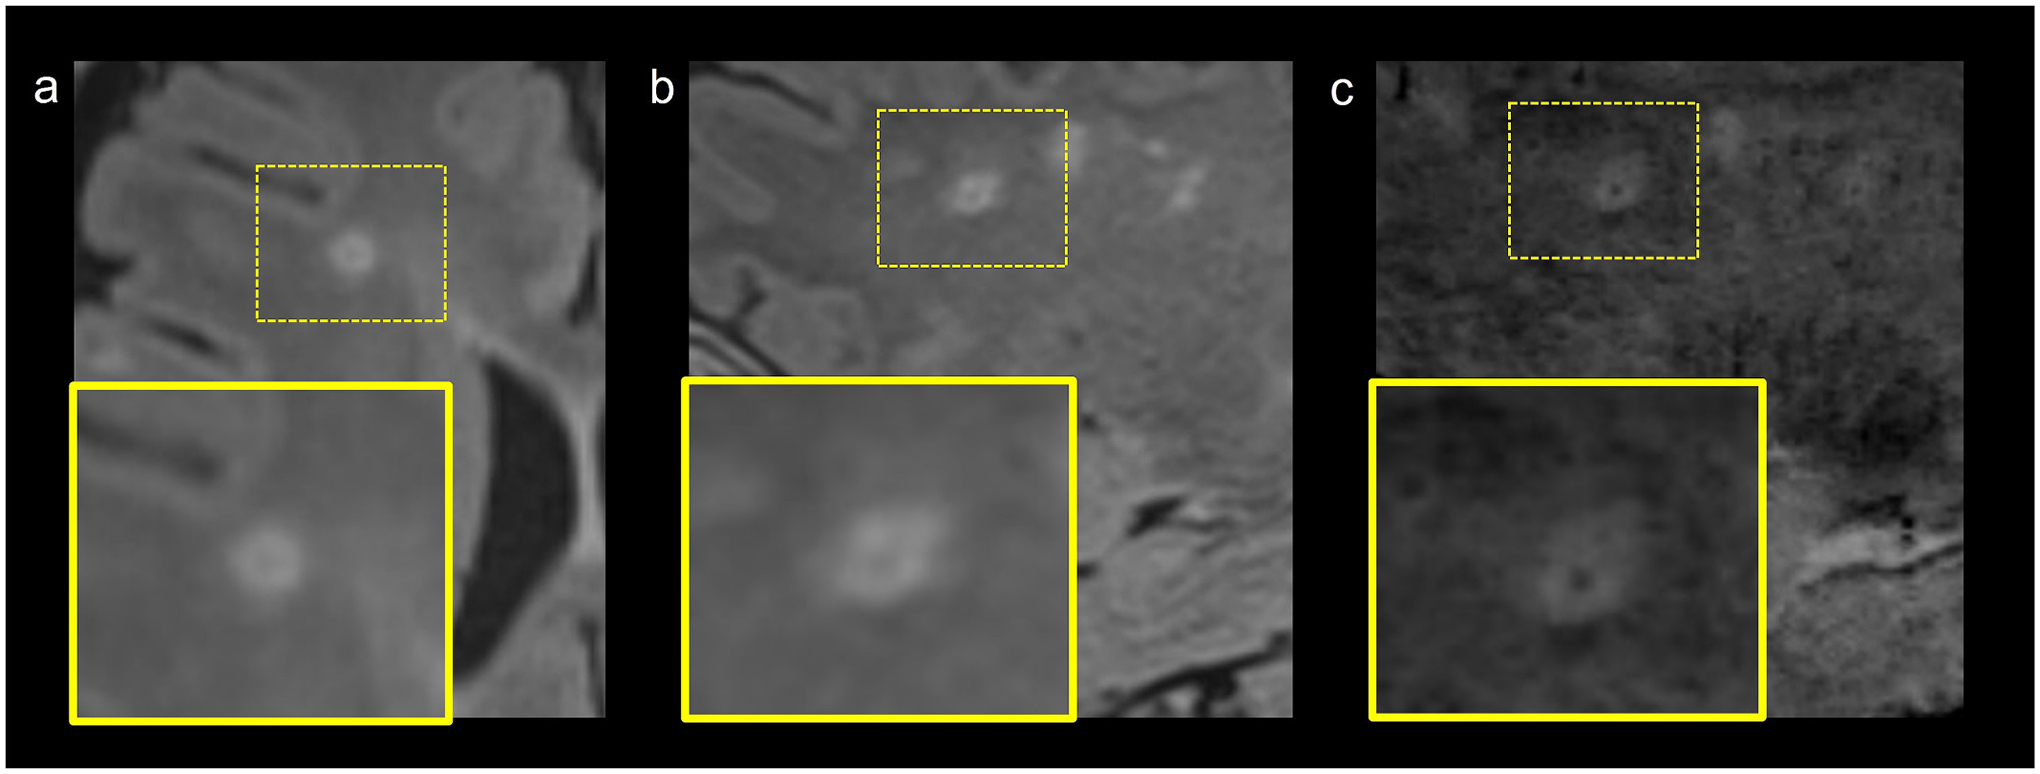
Scan from an individual with known diagnosis of multiple sclerosis obtained as part of routine clinical practice (Siemens Skyra 3 T). Axial (a) and sagittal (b) FLAIR showed a hyperintense lesion with a central hypointensity, with corresponding sagittal SWI (c) demonstrating the same.
